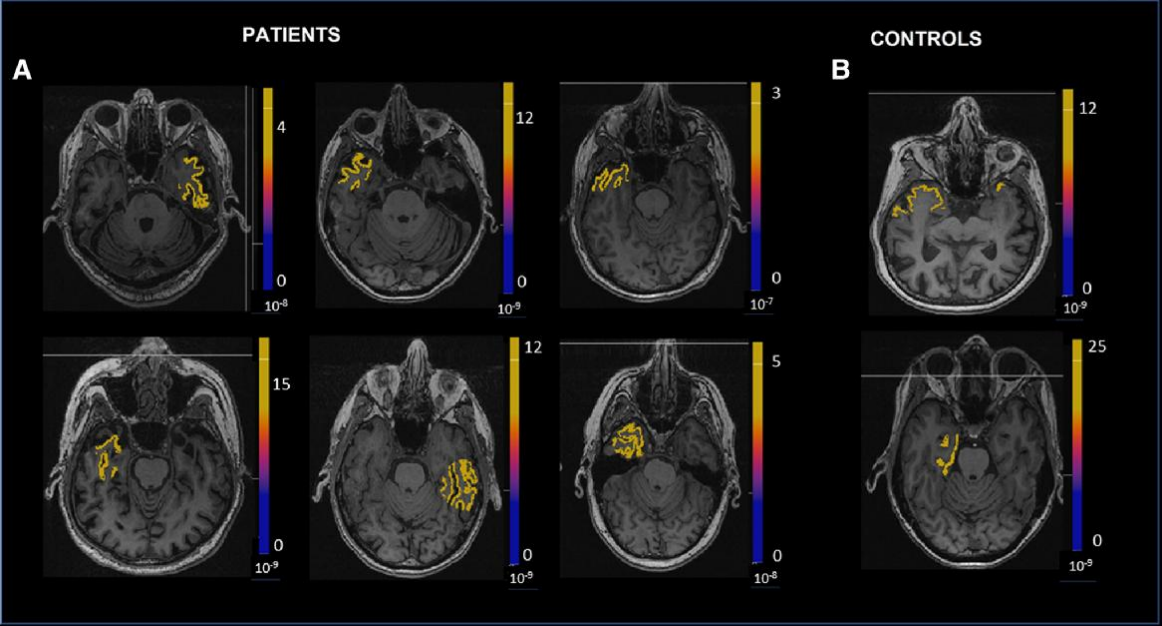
Electronic source imaging in patients and controls. Overview of sources at the time peak of one epileptiform discharge, shown on individual MRI viewer on axial view in (A) patients with aMCI and (B) HC.
